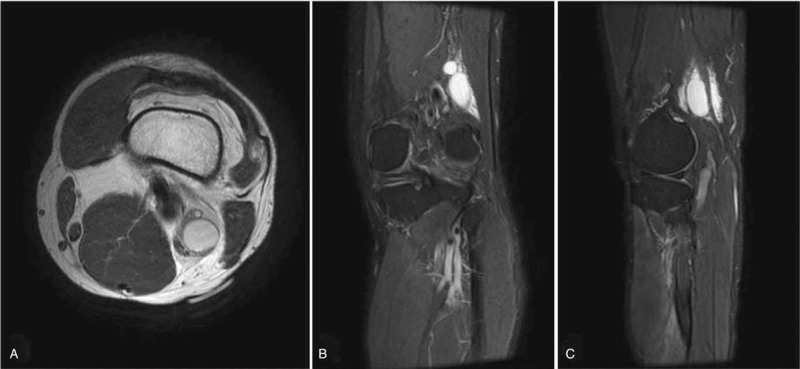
Axial (A), coronal (B), and sagittal (C) views of T2-weighted magnetic resonance images show an approximately 18 × 17 × 41 mm sized multilobulated cystic lesion, which was located anteromedial to the common peroneal nerve in the supracondylar area of the left femur.
